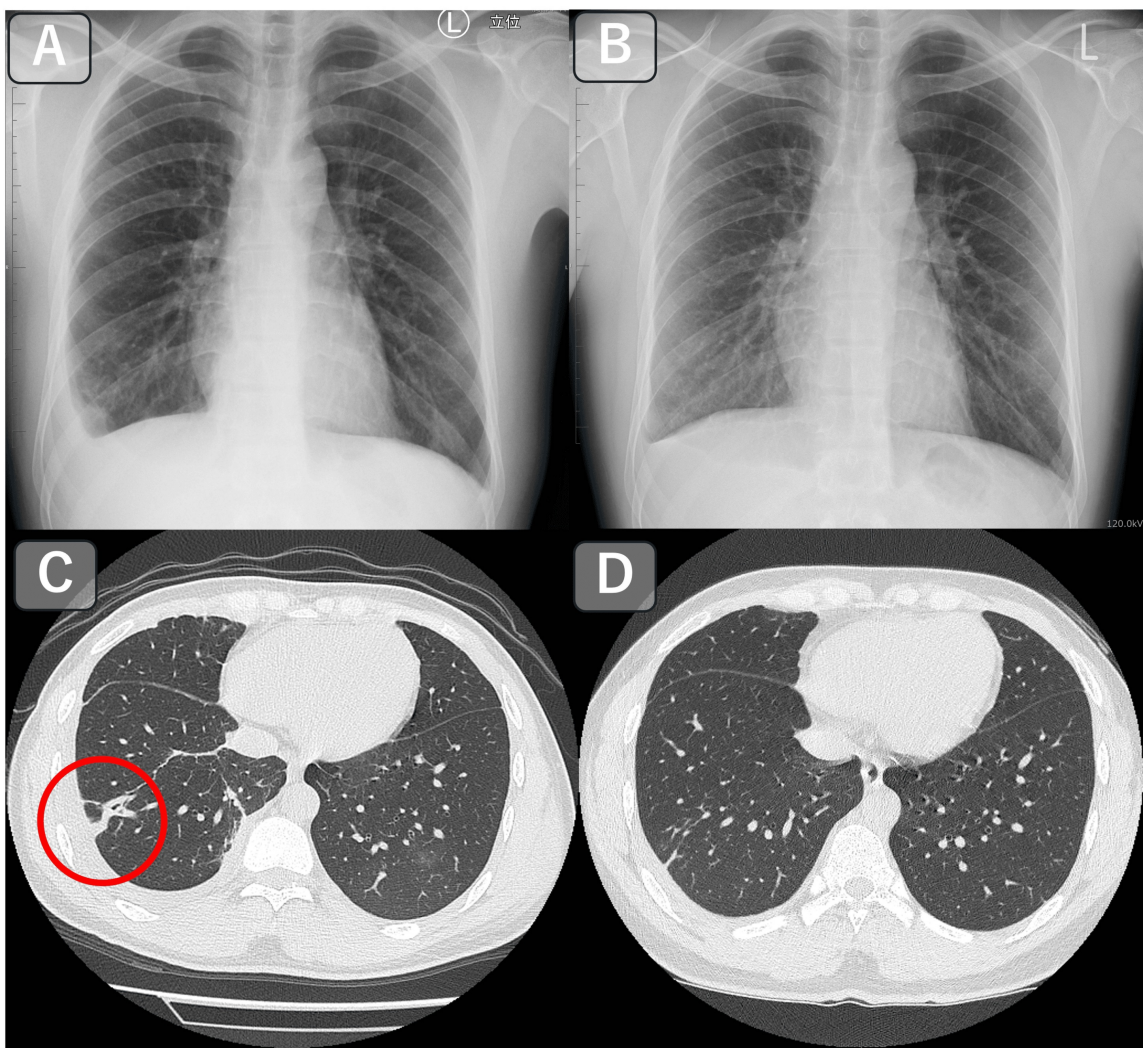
Radiographic and CT Findings Before and After Treatment. (A) Chest X-ray prior to treatment showing right-sided pleural effusion.
